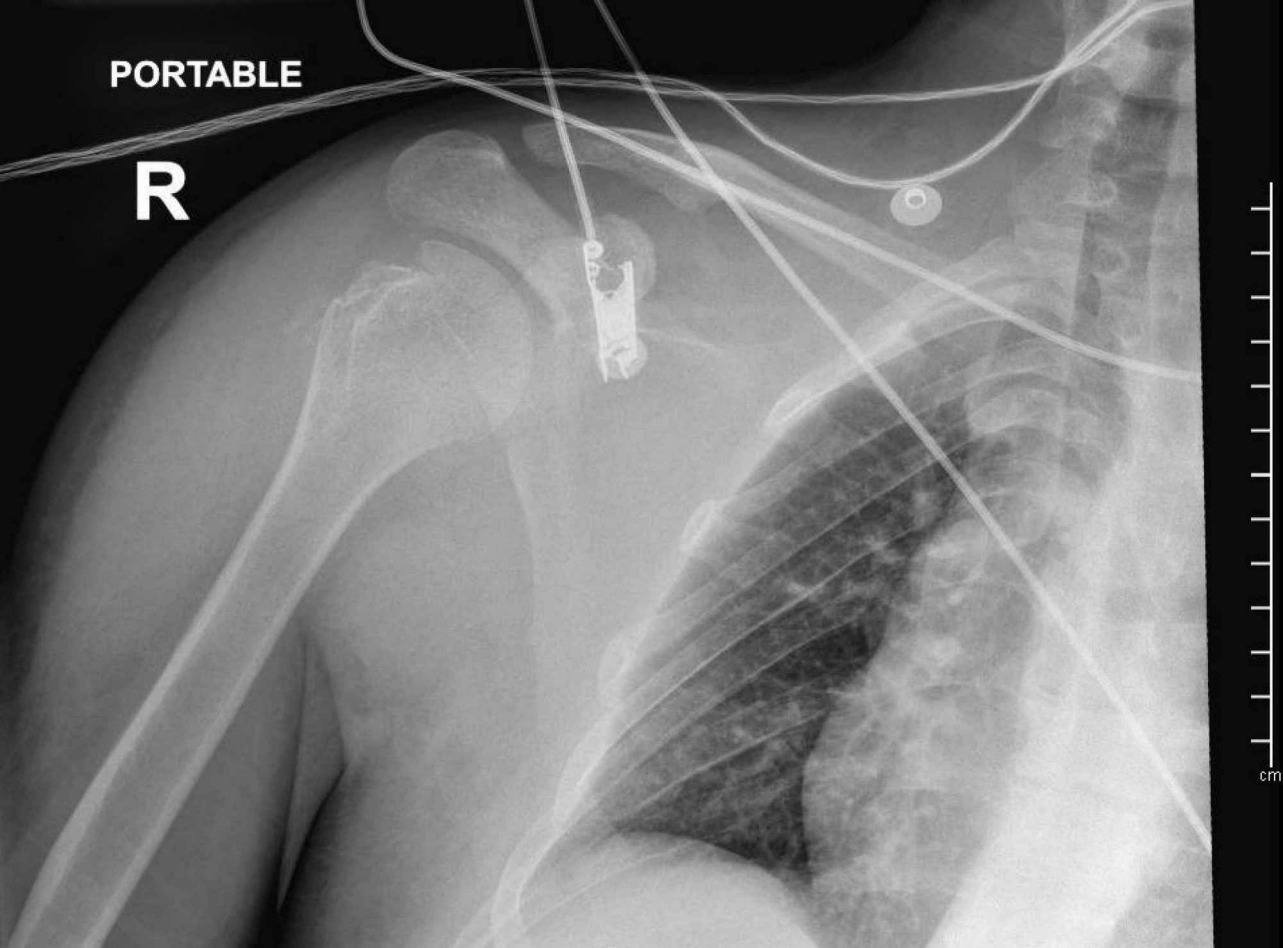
X-ray of the right shoulder after reduction.
